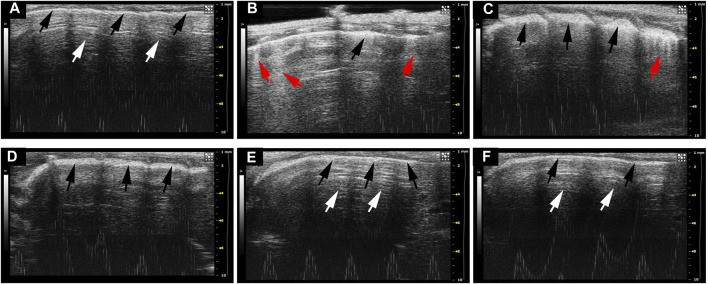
The ultrasound imaging lesions of ARDS were evaluated by lung ultrasound. (A) Control group: (B) LPS+PBS group; (C) LPS+2.6 g/kg NFPX group; (D) LPS+5.2 g/kg NFPX group; (E) LPS+10.4 g/kg NFPX group; (F) 10.4 g/kg NFPX group. White arrow: A-lines; red arrow: B-lines; black arrow: pleural line.
